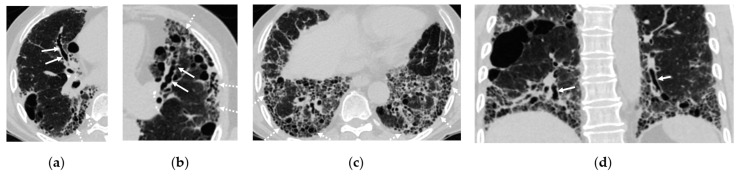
Interstitial lung disease found on a computed tomography (CT) scan of a 72-year-old male with hypertension, cough, and history of smoking. (a–c) Axial CT scans demonstrate traction bronchiectasis in right middle lobe (arrows in a) and left upper lobe (arrows in b) as well as reticulations and cystic changes at the periphery of both lungs (dashed arrows in a–c). (d) Coronal CT scan reveals traction bronchiectasis (arrows) and basilar predominance of the cystic change or honeycombing.
